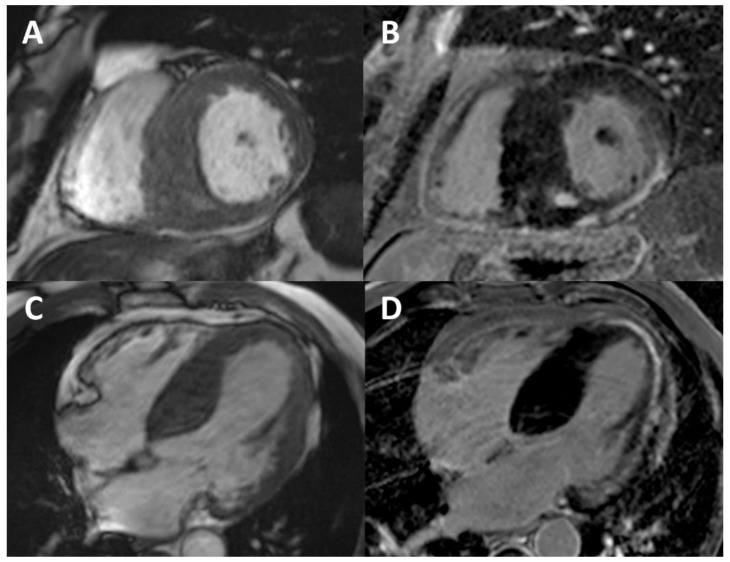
Cardiac MRI (3.0 Tesla) in a Fabry patient with advanced cardiomyopathy. Cine (balanced steady-state free precession sequence) images at the basal LV short-axis slice (A) and four-chamber view (C) showing massive and asymmetrical LVH (maximal thickness 30. mm at the septum) with thinning of the posterior wall (2 mm). LGE at the basal LV short-axis slice (B) and four-chamber view (D) showing fibrosis of the inferior and inferolateral walls and apex and focal fibrosis in the septum, where the LVH is more pronounced.
